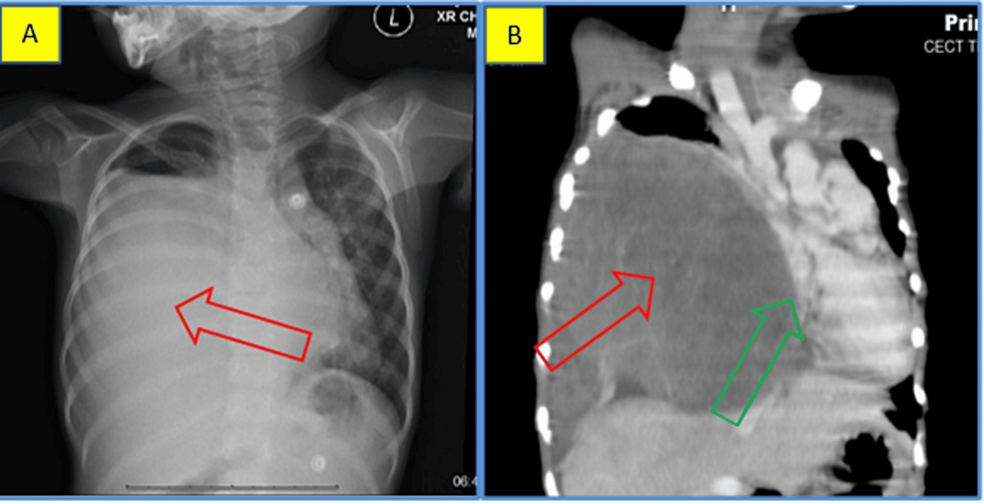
Case 2: radiological features A. Chest radiograph showing an opaque right hemithorax-sparing apex (red arrow) with mediastinal shift to the left side. B. Coronal reformation of CT shows the mass filling of the right lung (red arrow) and pleural space, displacing the heart to the left side (green arrow).
